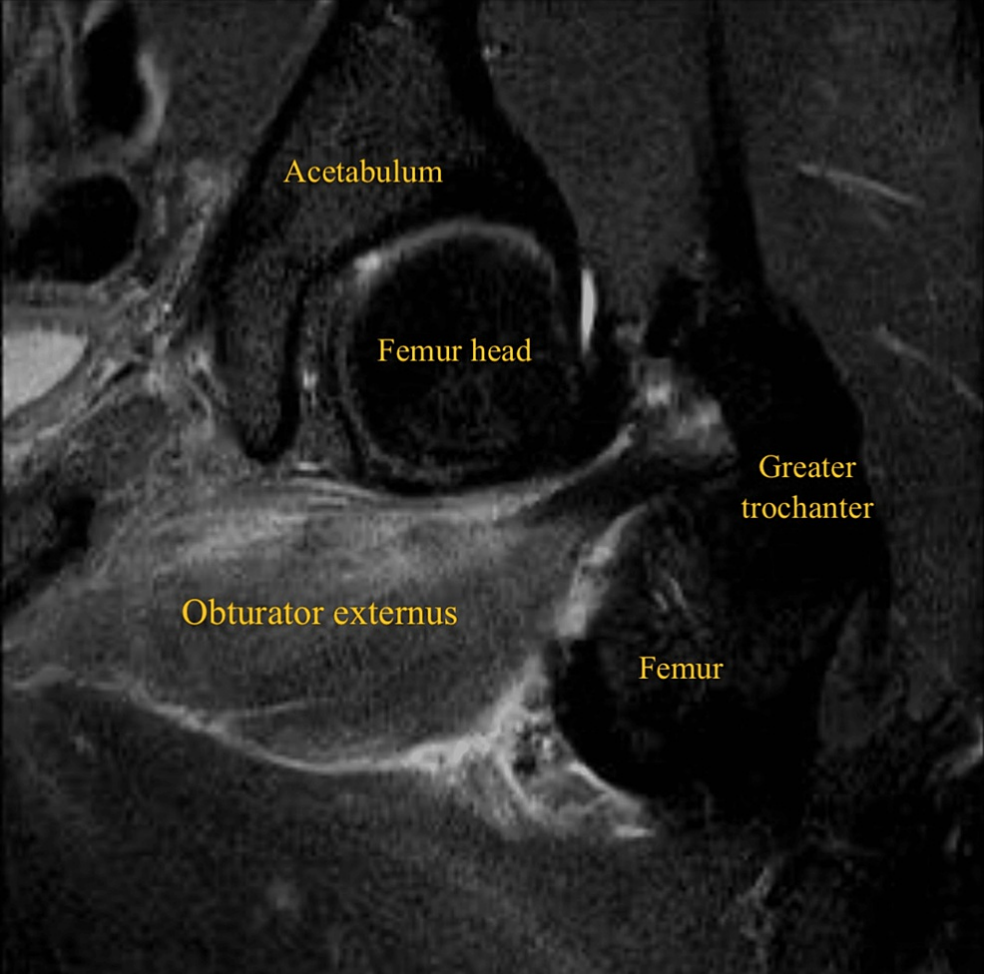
Coronal oblique short-tau inversion recovery (STIR) MRI of the left thigh showing left obturator externus tear
